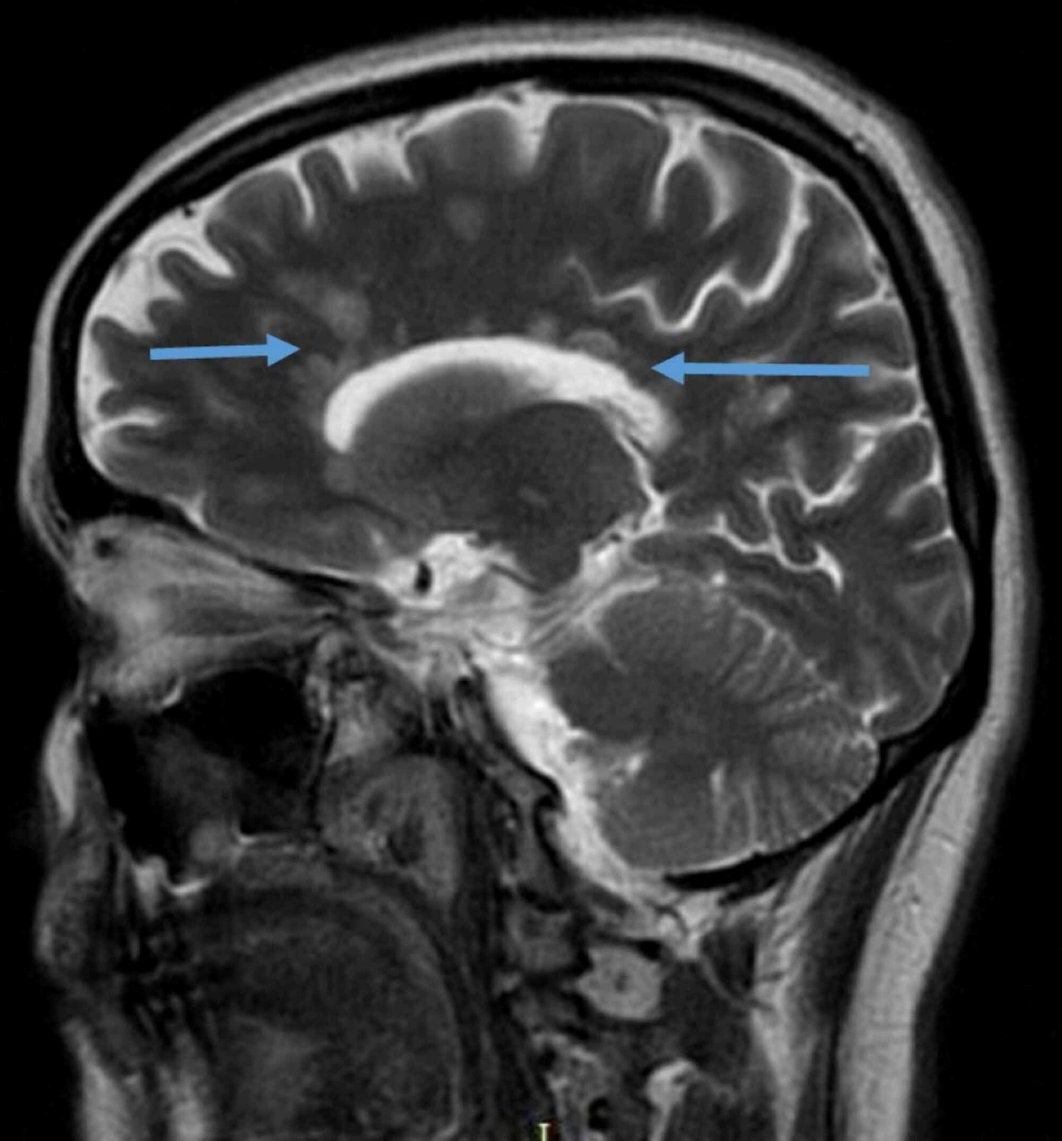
Coronal FLAIR T-2 weighted image of the patient’s brain demonstrating multiple periventricular plaques with long axis perpendicular to the corpus callosum (blue arrows). FLAIR: Fluid attenuated inversion recovery.
